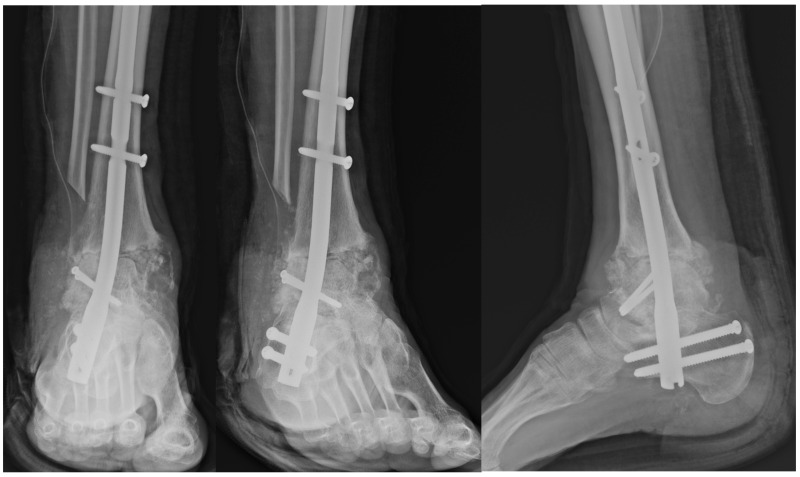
Postoperative radiograph demonstrating fixation of the talar allograft to the residual talar head using two 3.5 mm cannulated screws for structural stability.
